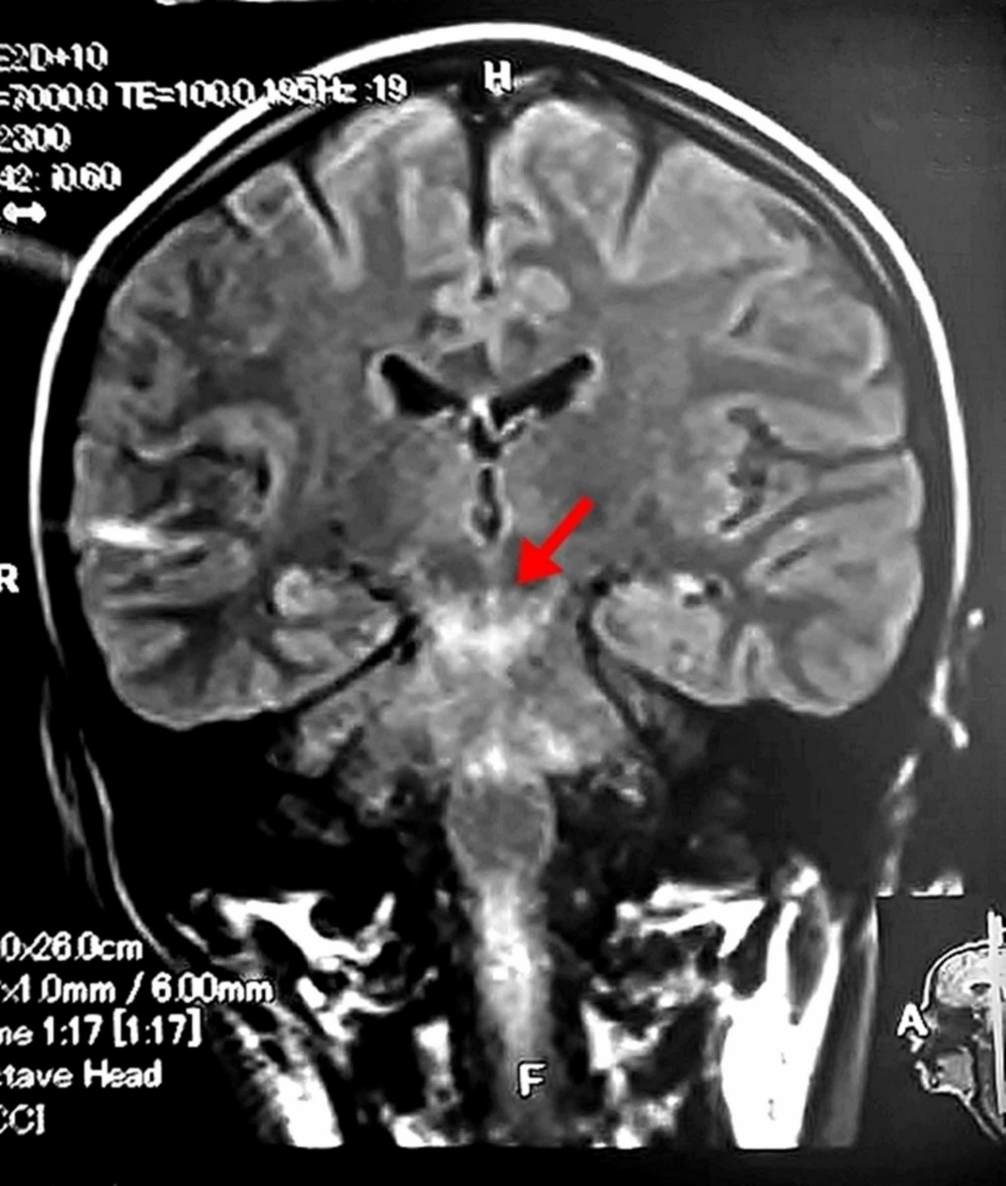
MRI of the brain showing abnormal signal intensity changes seen in the midbrain and pons extending into the periaqueductal region
